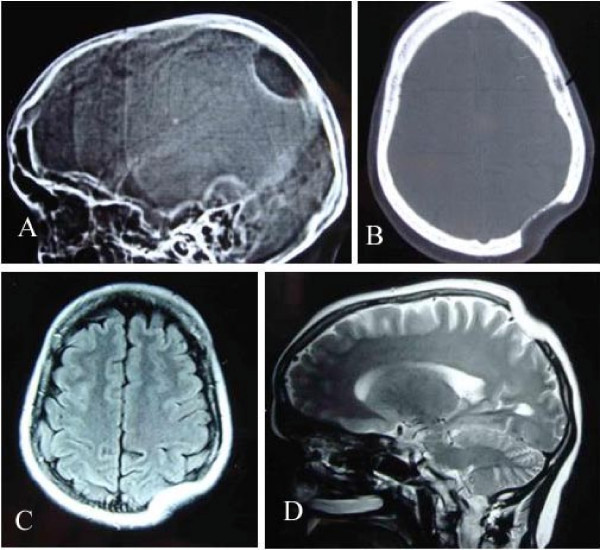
X-ray skull lateral view (A) showing a osteolytic area in left parietal region. CT scan bony window (B), MRI T1W Axial (C) and T2W Sagittal (D) revealing skull defect with normal brain parenchyma.
